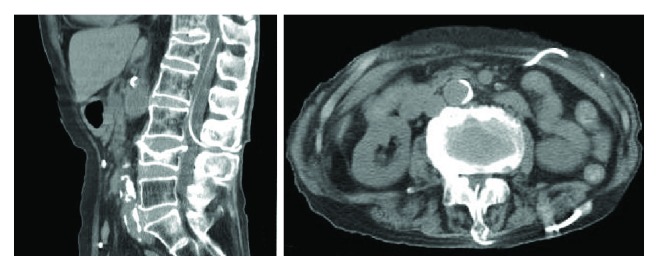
CT showed that LP shunt was routed from the subarachnoid space at the level of fourth and fifth lumber spines through the subcutaneous tissue of the left back and into the abdominal cavity.
